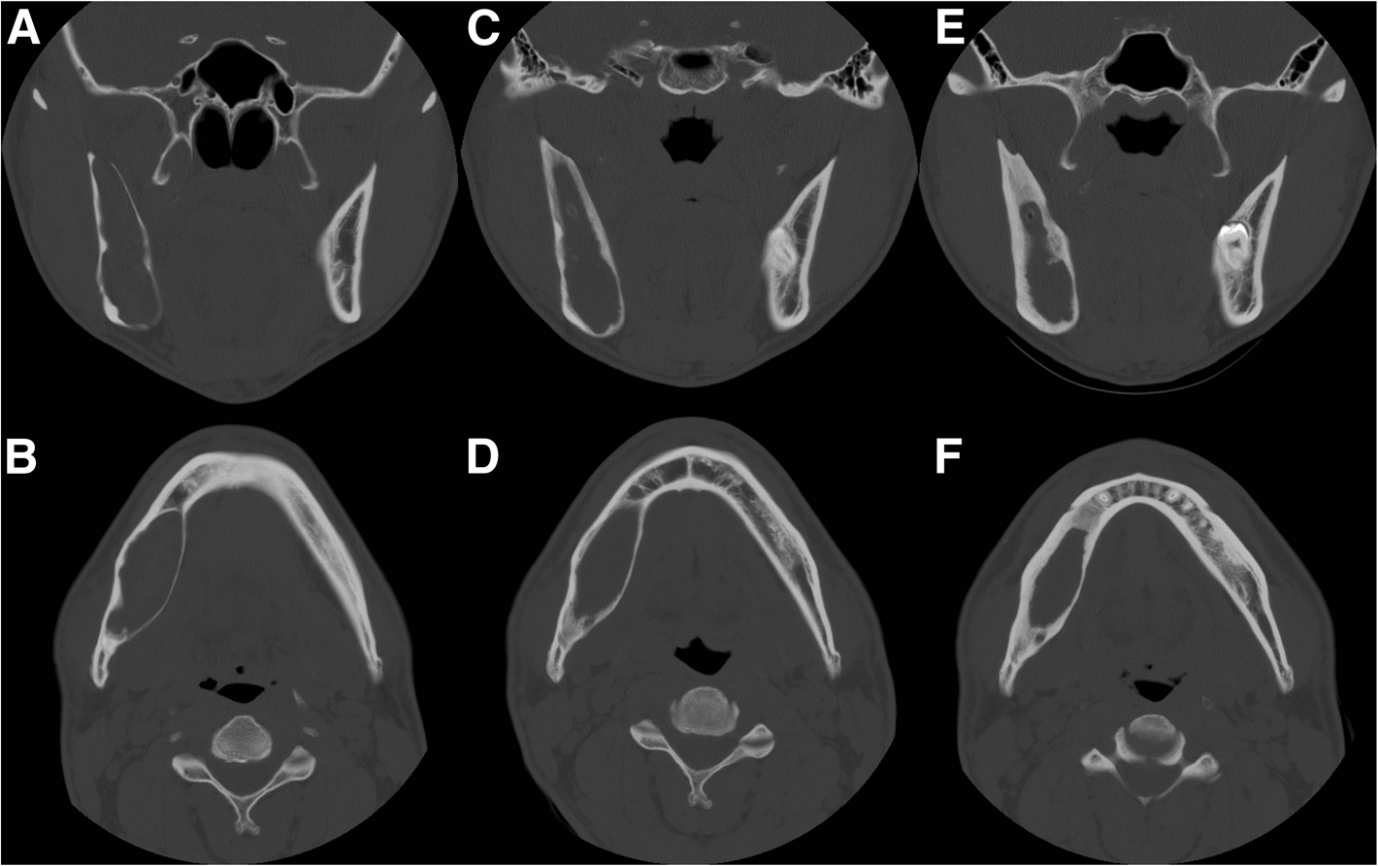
Representive serial axial and coronal CT of OKC decompression. During the decompression for about 18 months, remarkable reduction in lesion size and increased cortical bone thickness with new bone formation is observed. (a, b initial, c, d 6 months decompression, e, f 18 months decompression)
